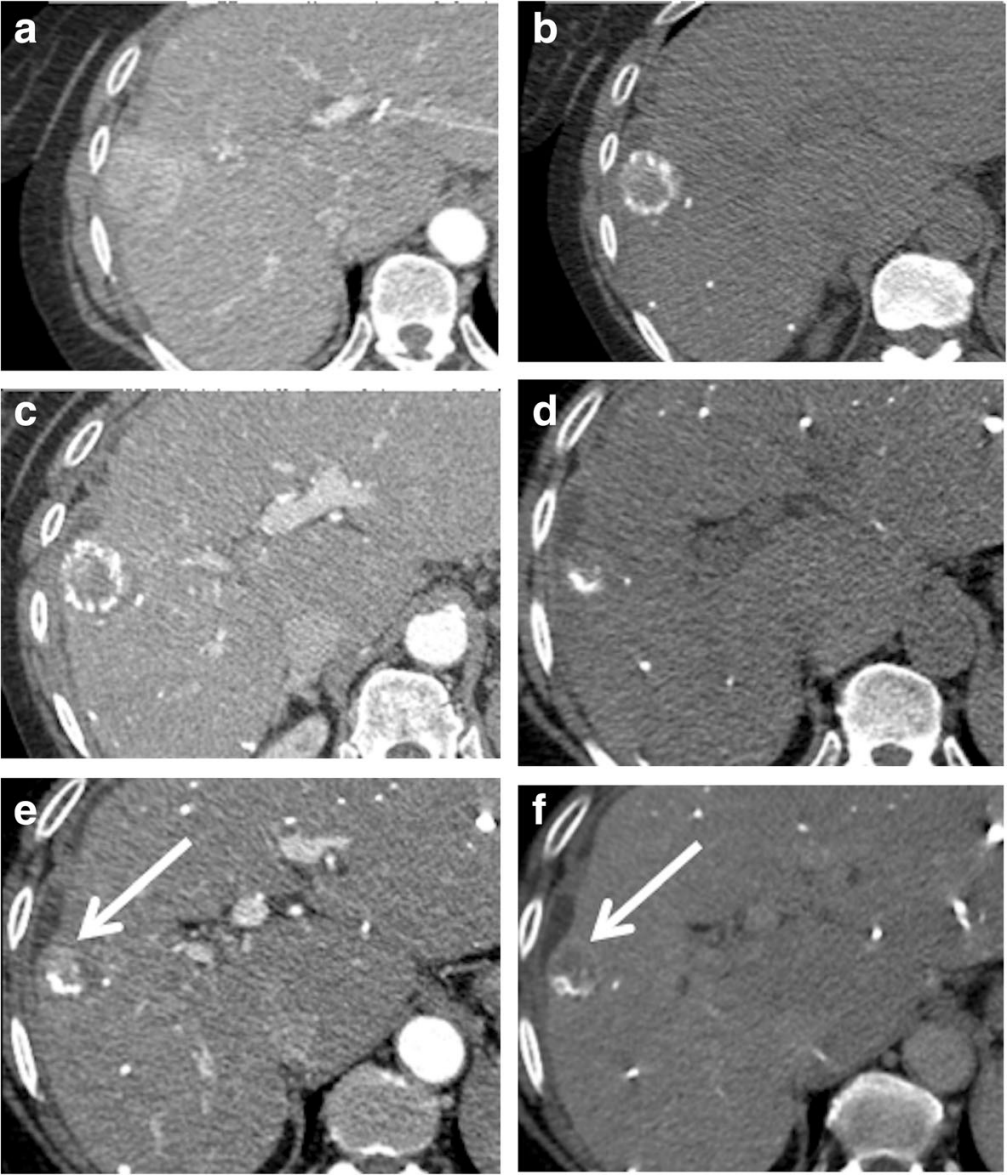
Local progression of an HCC showing a complete response according to mRECIST with incomplete lipiodol retention in a 59 yr-old female with HCV related cirrhosis. a baseline CT image obtained during hepatic arterial phase (HAP) shows a hyperehanced HCC in the right liver lobe. b and c first follow-up CT images showing the lesion presenting with incomplete lipiodol retention on a precontrast image (b), while images obtained during HAP (c) show no residual enhancement of the lesion. Millimetric lipiodol depositions were depicted in the right liver, suggesting the presence of pre-existing yet undetected small tumor foci. This CT showed the appearance of a lesion in the left liver lobe (not shown in the figure), for which the patient underwent a second TACE session targeting only the left liver lobe. d-f 3 months follow-up CT images obtained during precontrast (d), HAP (e) and delayed (3′) phase (f) show the reappearance of a hyperenhancing nodular portion on HAP (e) with washout on delayed (3′) phase (f) consistent with local progression (arrow)
